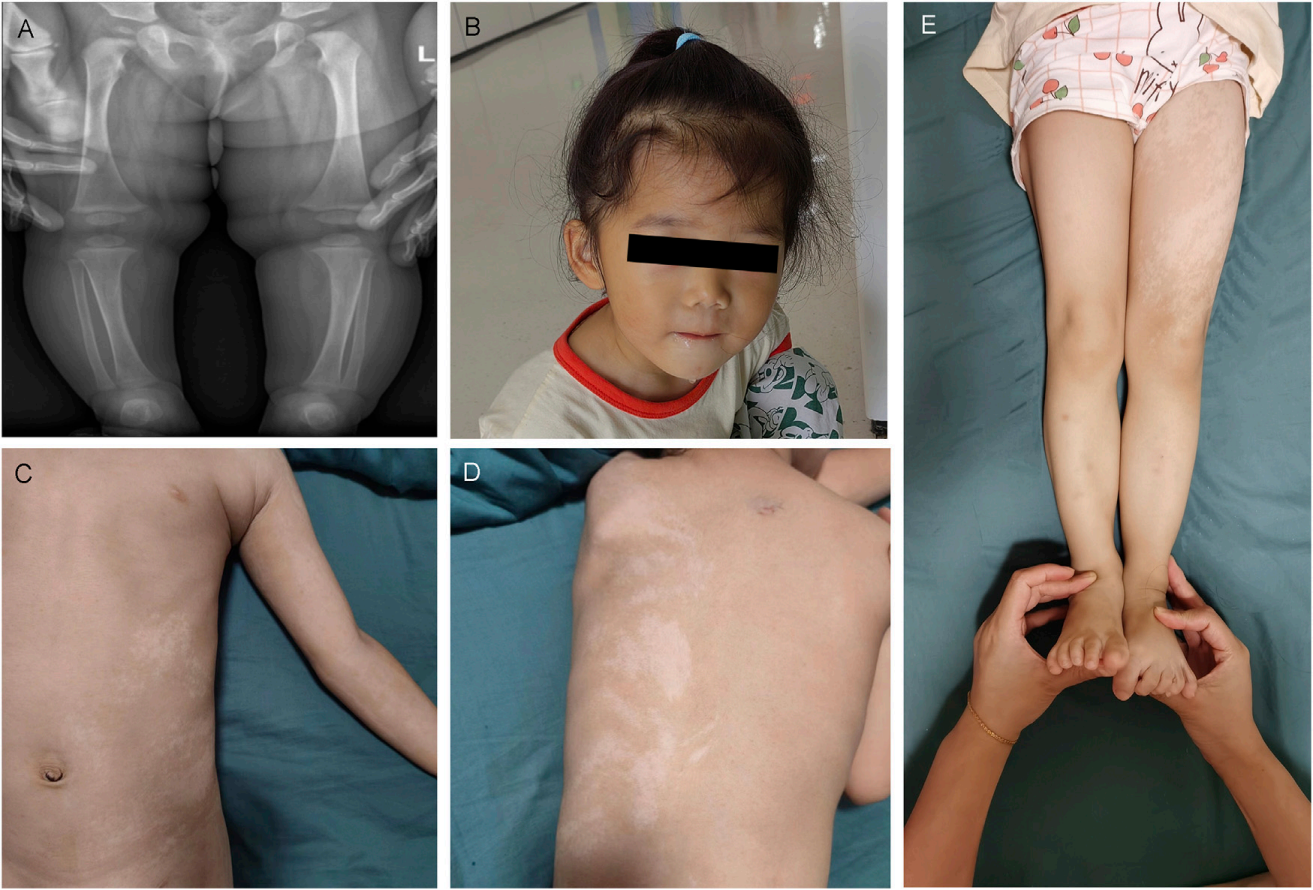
Clinical and imaging features of DII:1. (A) X-rays of the lower extremities of DII:1 showed unequal lengths and thicknesses of both her lower extremities without significant bone abnormalities. (B) DII:1 facial dysmorphic features, including high forehead, ocular hypertelorism, and low nasal bridge. (C–E) The loss of pigmentation within her skin, together with her longer and thicker left leg compared to her right leg.
